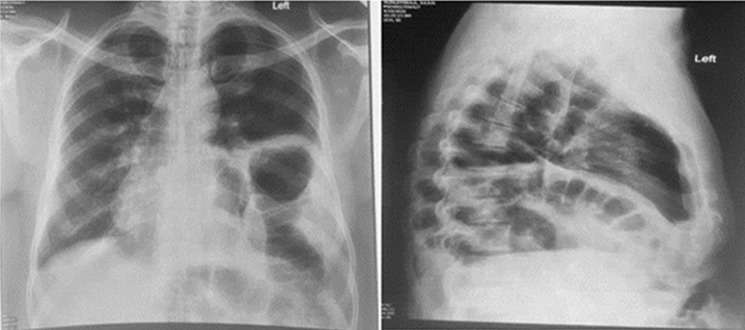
anteroposterior and lateral chest radiographs showing thinned out and markedly elevated left hemidiaphragm (diaphragmatic eventration) with mass effect of the sub-phrenic abdominal contents causing ipsilateral left lung collapse and contralateral trachea-mediastinal shift (tension viscero-thorax)
